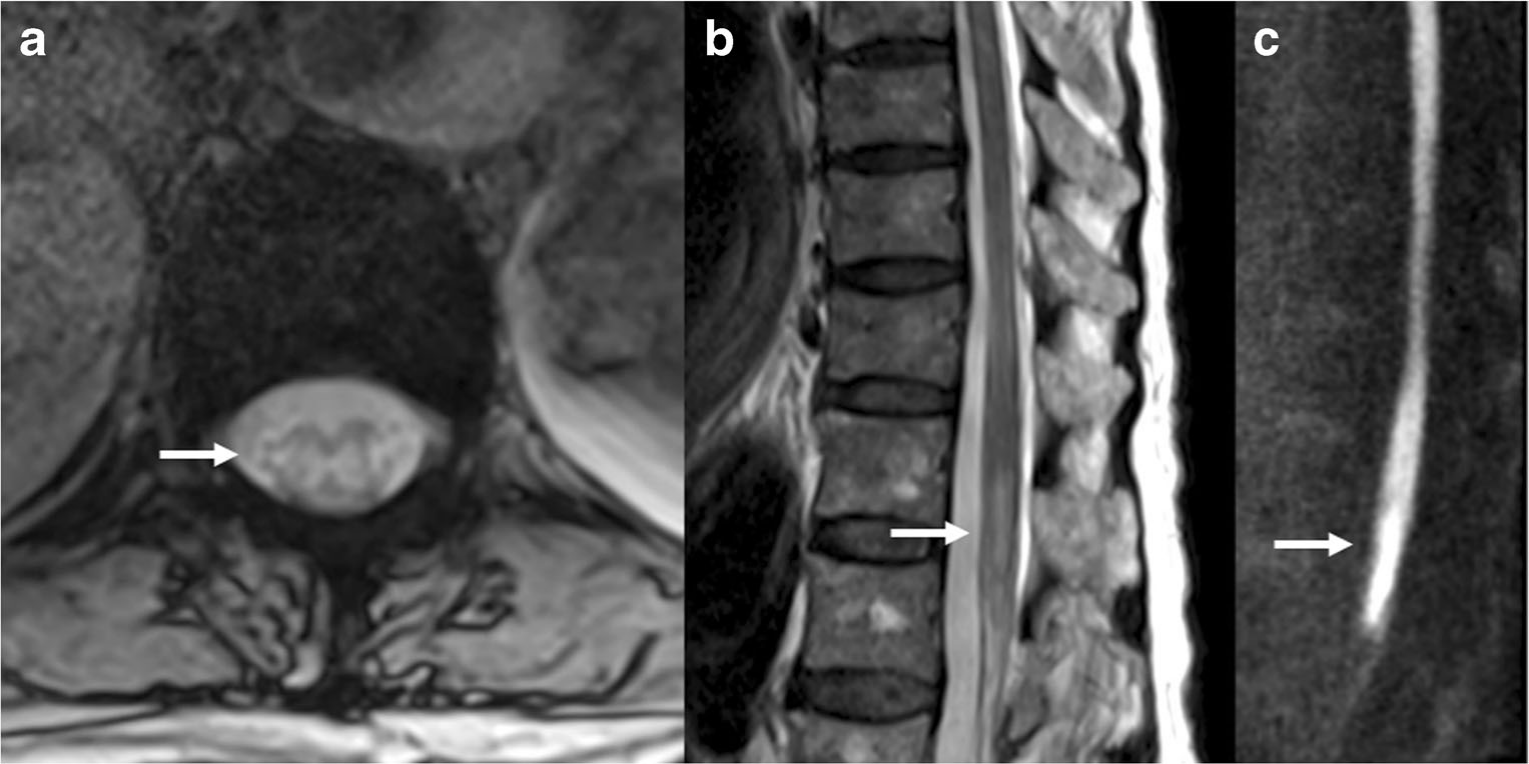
Spinal cord ischaemia. High T2 signal abnormality in the conus medullaris with diffusion restriction on DWI (arrows) compatible with an ischaemic lesion
